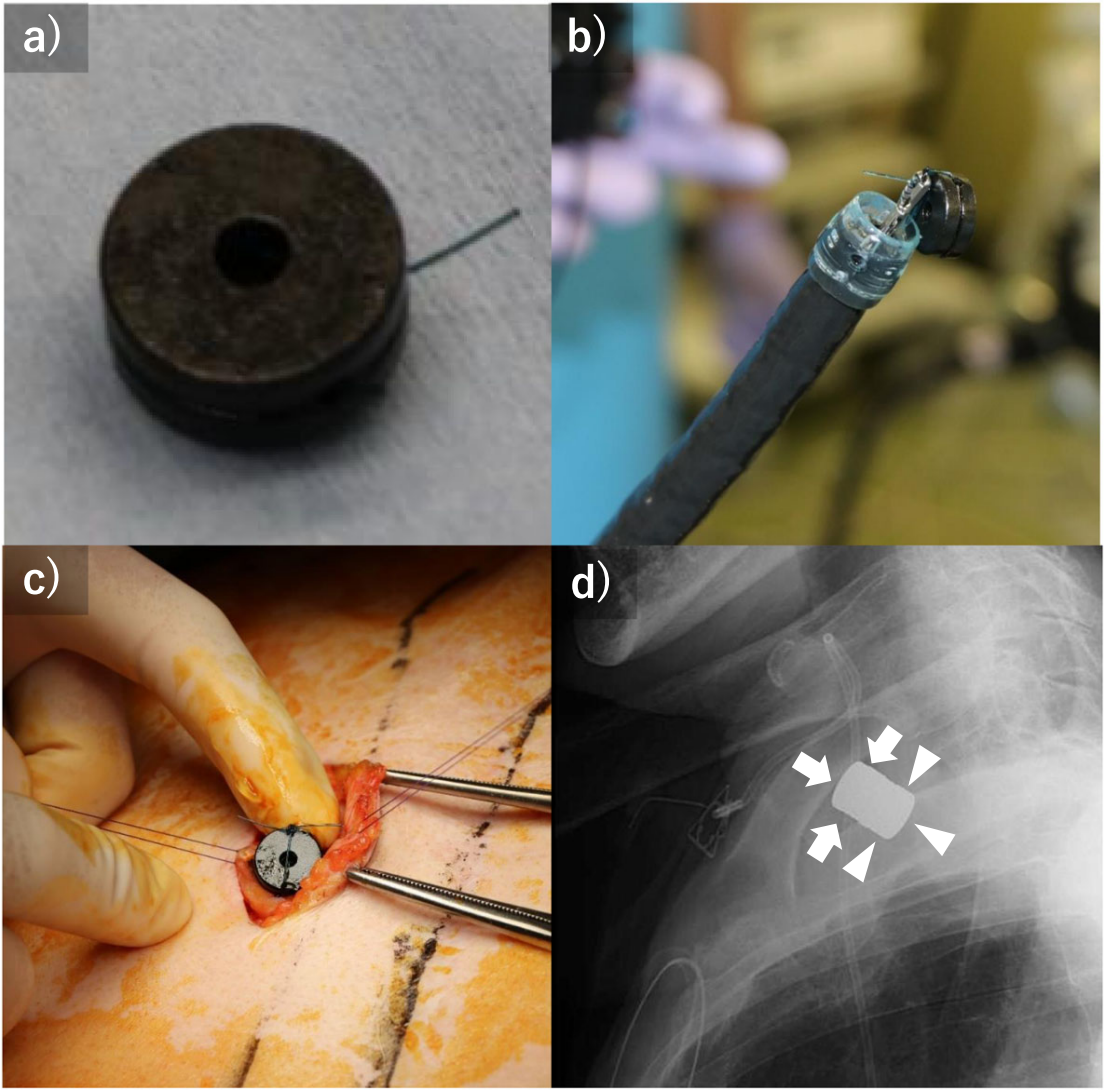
a A magnet of 12.5 mm in diameter, 5 mm in thickness, and 3500 gauss was used. b A magnet was placed on the left esophagus side using an endoscope. c The magnet was inserted directly into the blind end of the colon. d It was confirmed by X-ray examination that the magnets left in place were attracted (arrows are the magnets of the left esophagus side; arrow heads are the magnets of the colon side)
